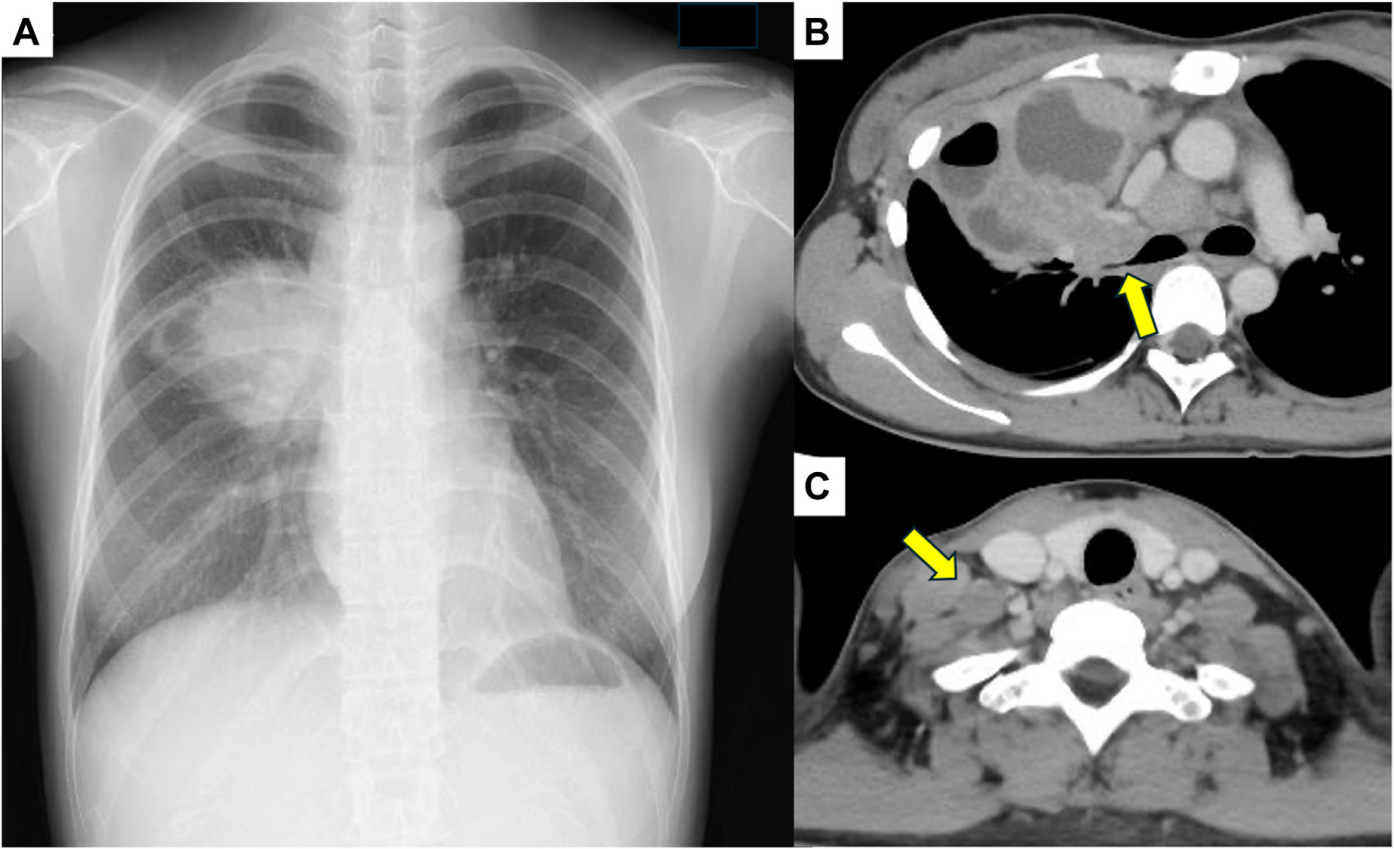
Initial imaging findings. (A) Chest radiography revealed consolidation in the right upper lung field. (B) Chest computed tomography demonstrated a cavitary lesion with an air-fluid level in the right upper lobe. The right upper lobe bronchus showed compressive stenosis caused by lymphadenopathy (yellow arrow). (C) Right supraclavicular lymphadenopathy was observed (yellow arrow).
